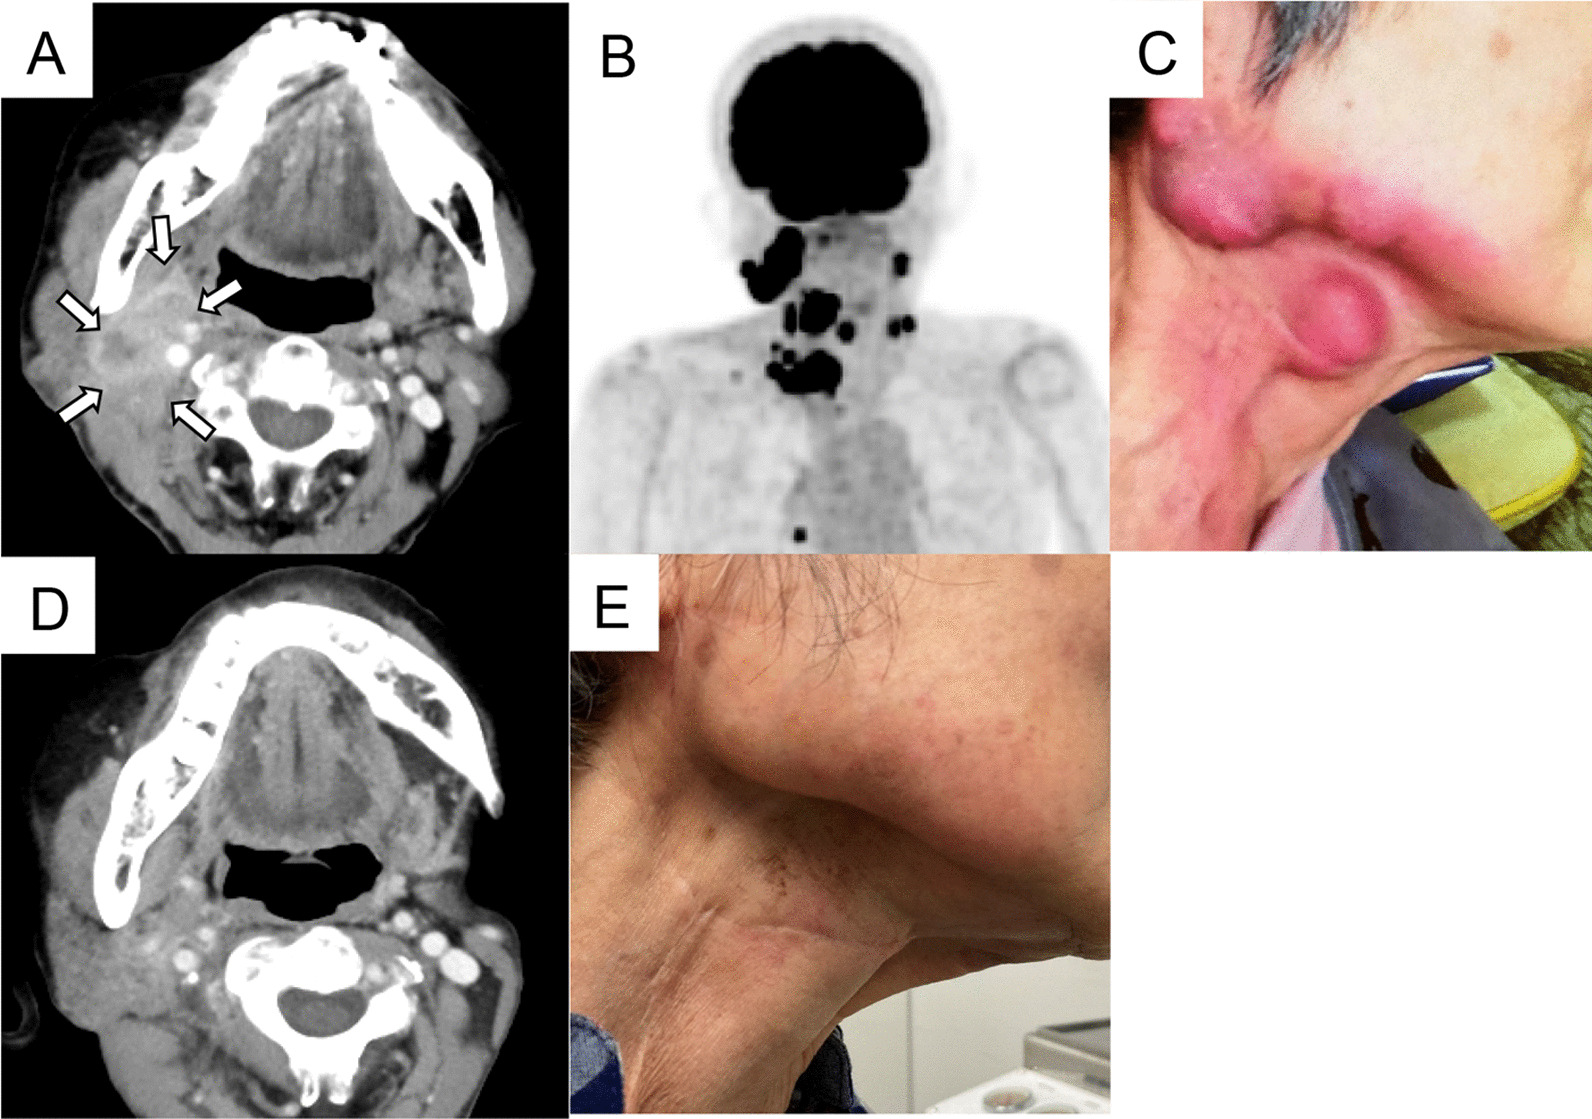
Computed tomography (CT) and positron emission tomography-computed tomography (PET-CT) course. Two months after right cervical dissection for right submandibular lymph node metastasis, computed tomography revealed right cervical recurrence and lung metastasis (A), positron emission tomography-computed tomography demonstrated multiple cervical metastases (B), and skin erythema associated with tumor invasion (C). Five months after initiation of pembrolizumab therapy, computed tomography revealed marked shrinkage of the tumor (D), which was evaluated as complete remission (CR), and skin erythema was absent (E)
